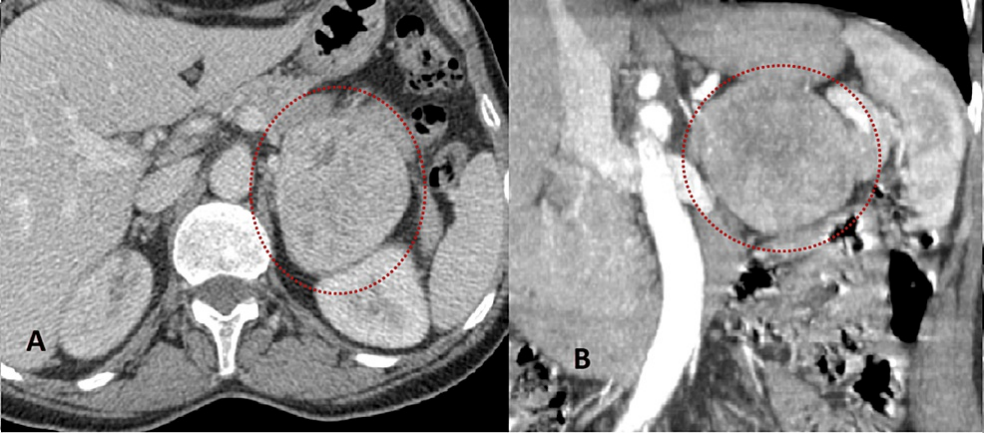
Coronal (A) and axial (B) images of abdominal CT, in arterial (A) and portal venous phases (B). Left adrenal lesion (red circles), measuring 7 cm × 6.5 cm, with rounded morphology and well-defined margins, with heterogenous and progressive enhancement in, with internal ill-defined hypoenhancing areas.
